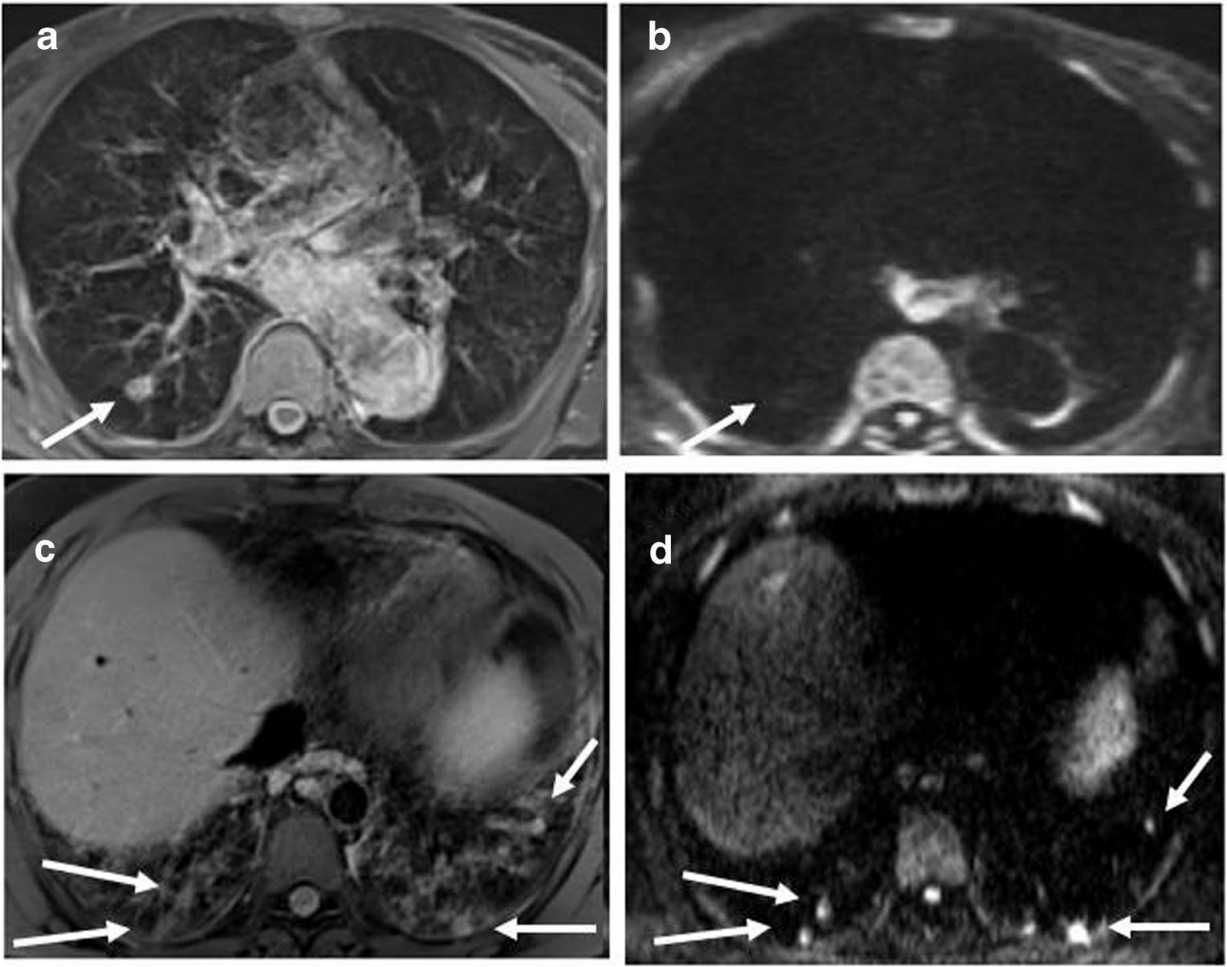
Representative MRI with DWI sequences: nodules Nodules detected by MRI with DWI. MRI BLADE sequence detecting a nodule (*white arrow*) at the right lower lobe (a) without a DWI corresponding hotspot (b). MRI BLADE sequence detecting multiple and bilateral small nodules of the lower lobes (c) and their corresponding hotspots at DWI assessment (*white arrow*) (d)
